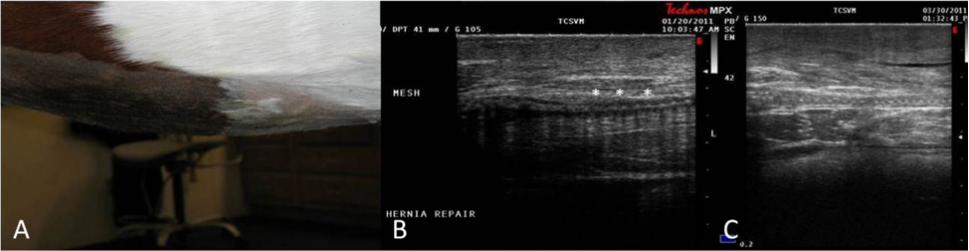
Postoperative (8-week) image of the ventral midline following mesh hernioplasty (A) with corresponding ultrasound images (B, C) documenting soft tissue incorporation surrounding the silk mesh. Hypoechogenic shadow artifacts (*) representing the silk mesh are evident. Measurement markings on right side of ultrasound images indicate 0.5-cm intervals.
